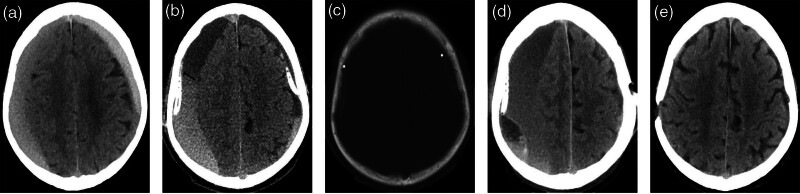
Axial CT scans of case 1. (A) Preoperative image; (B) a linear high-density lesion (black arrow) at the anterior edge of the drainage tube suggests outer membrane detachment and bleeding at 12 h postoperatively; (C) a bone window showing the drainage tube closely adhered to the skull; (D) increased right-sided subdural hematoma at 56 h postoperatively; (E) resolution of cSDH and return to neurologic baseline at 69 d postoperatively. cSDH = chronic subdural hematoma, CT = computed tomography.
